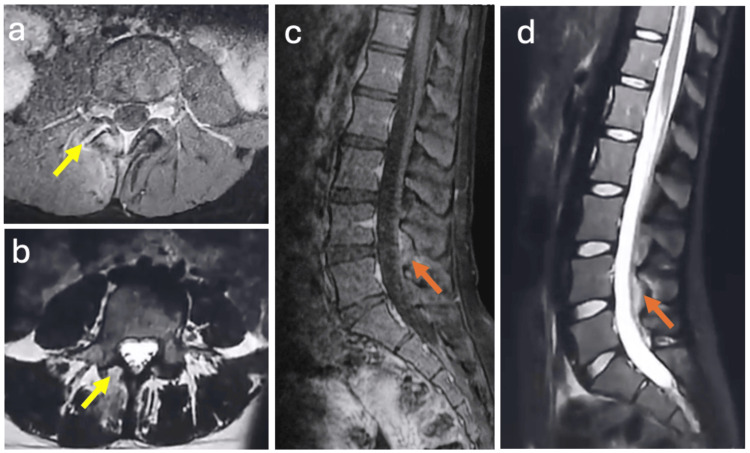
One-month follow-up MRI of the lumbosacral spine (Case 2). Axial fat-suppressed contrast-enhanced T1-weighted image (a) demonstrates persistent enhancement of the right L4-L5 facet joint (yellow arrow) without residual epidural collection. Axial T2-weighted image (b) shows hyperintense inflammatory signal changes involving the left inferior articular process of L4 (yellow arrow). Sagittal contrast-enhanced T1-weighted image (c) and sagittal T2-weighted image (d) demonstrate near-complete resolution of the epidural abscess, with residual inflammatory changes in the ligamentum flavum and interspinous soft tissues (orange arrow). These findings demonstrate radiological lag behind clinical improvement, with persistent MRI abnormalities despite complete clinical recovery. MRI, magnetic resonance imaging
